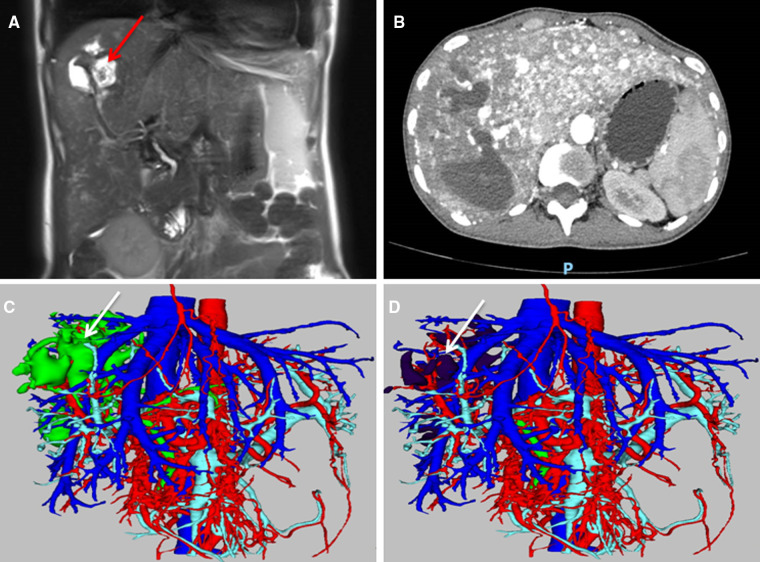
Imaging of HHT involvement in the liver. (A) The MRI results suggested right hepatobiliary dilatation with stone formation (arrow); (B) The arterial phase of CT indicated diffuse telangiectasia and shunting in the liver; (C) Three-dimensional reconstruction showed hepatic telangiectasia and bile duct dilatation (arrow); (D) Three-dimensional reconstruction showed telangiectasia and intrahepatic bile duct stones (arrow).
